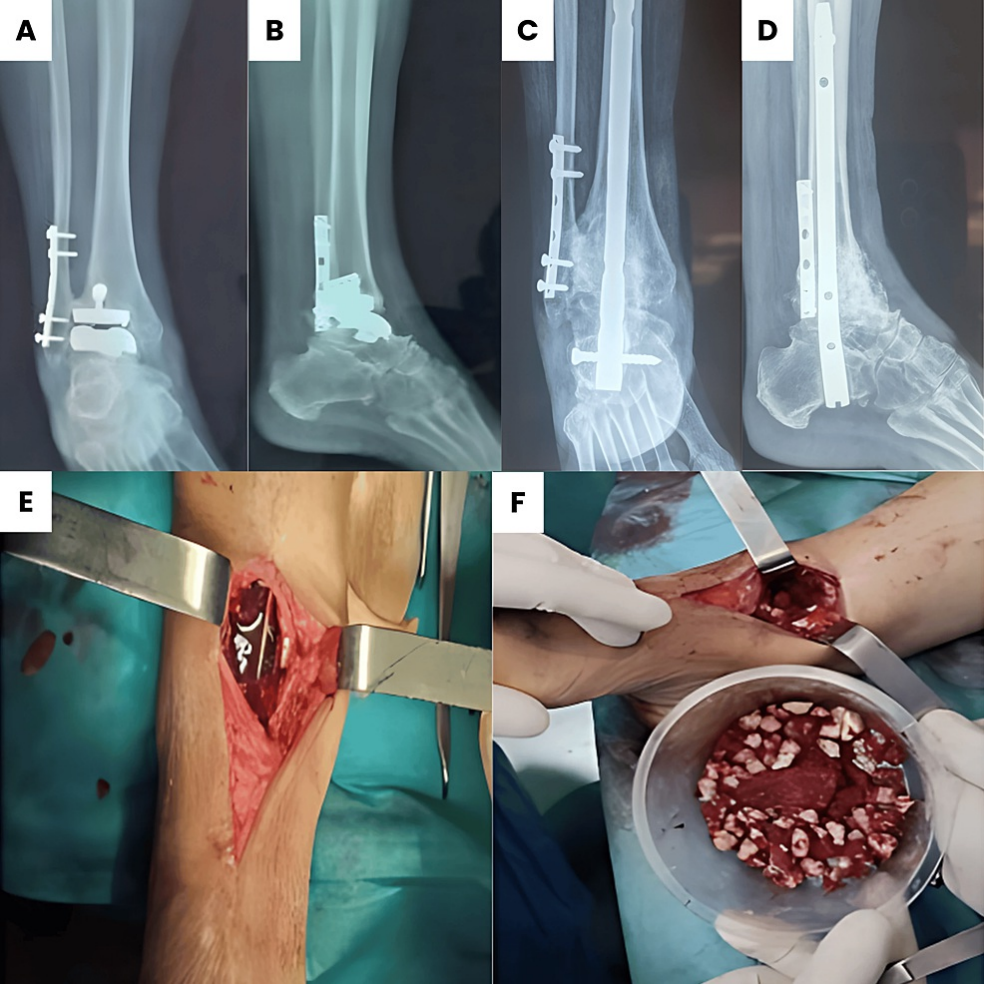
Management of loose total hip prostheses. Antero-posterior (A) and lateral (B) X-rays of the ankle show a loose TKA. Autograft and allograft to fill massive bone loss after TKA removal (E, F). Antero-posterior (C) and lateral (D) X-rays of the ankle 16 months after TTCA with retrograde femoral nail.
